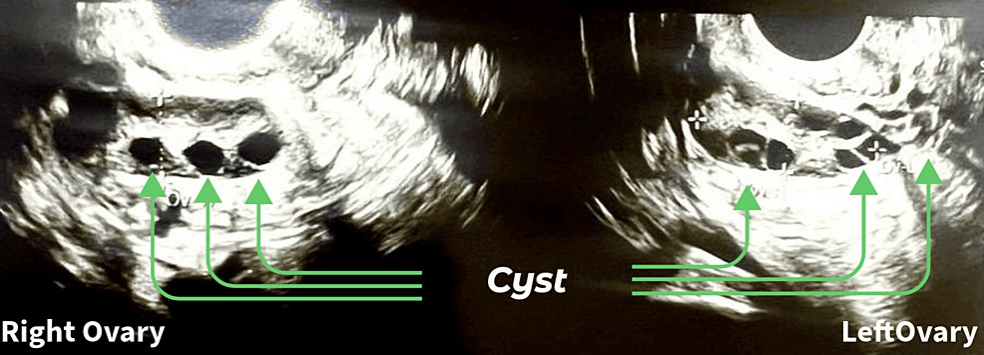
USG of female partner showing multiple cysts USG: ultrasound sonography
